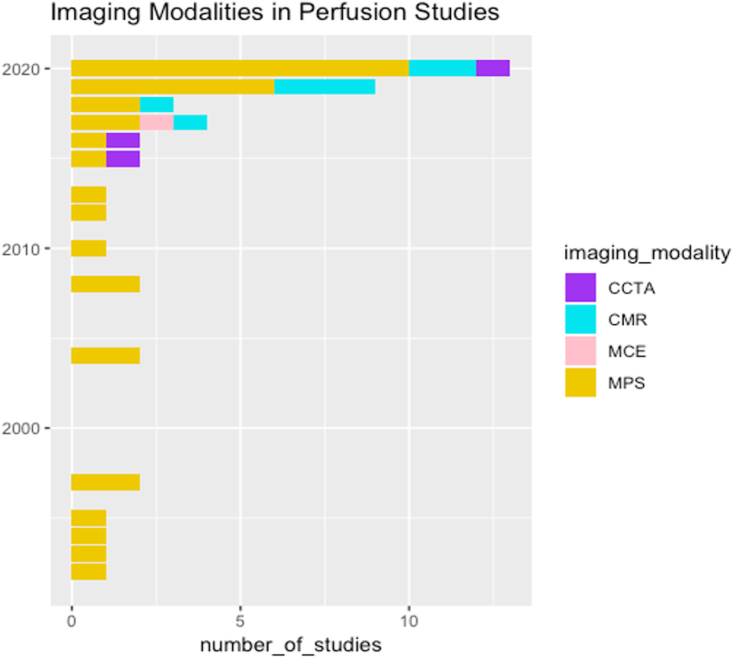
A stacked barplot showing the number of publications for each imaging modality in myocardial perfusion imaging over the last 30 years. CCTA, coronary computed tomography angiography; CMR, cardiac magnetic resonance; MCE, myocardial contrast echocardiography; MPS; myocardial perfusion scintigraphy.
